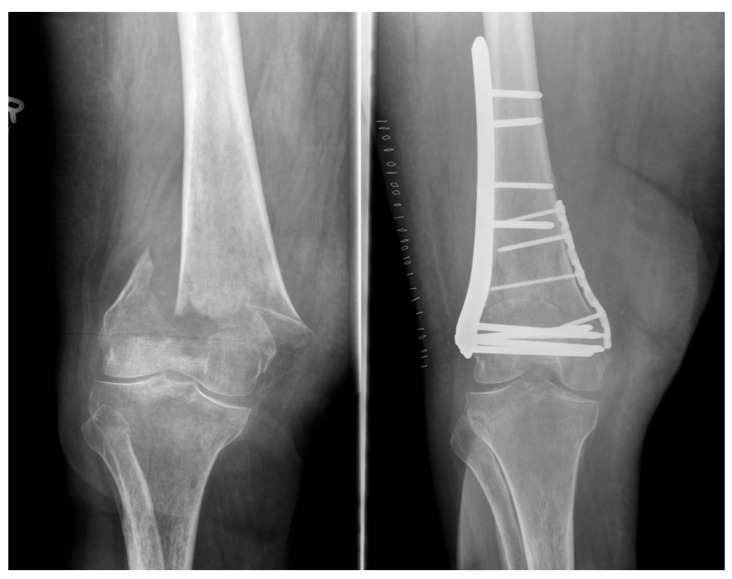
Radiographs of a 56-year-old female patient with an osteoporotic DFF treated with double-plating osteosynthesis.
